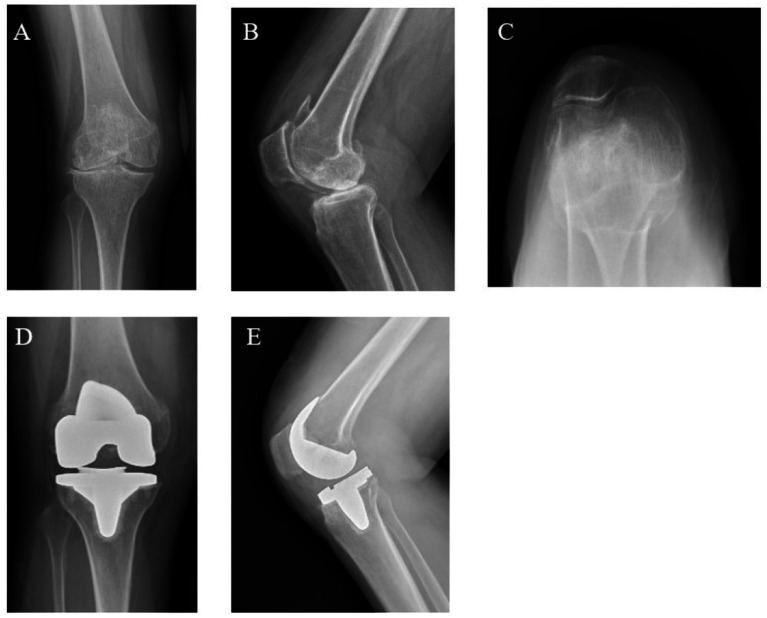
Representative radiographs of a patient treated with TKA. (A–C) Preoperative AP, lateral, and skyline (axial) views showing predominant lateral compartment osteoarthritis with preserved medial joint space and mild-to-moderate patellofemoral degeneration. (D,E) Postoperative AP and lateral views demonstrating implanted components on follow-up radiographs.
